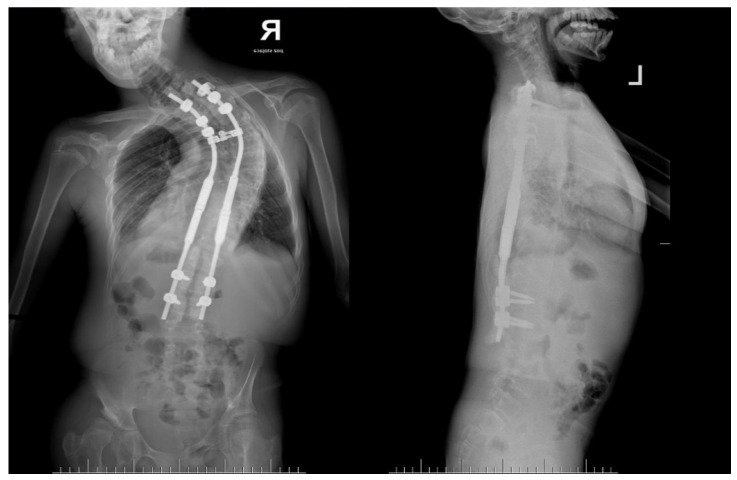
Radiological standing X-rays AP and LAT of 14-year-old boy at 1 year of follow-up showed correction of main curve from 130 degrees to 80 degrees.
